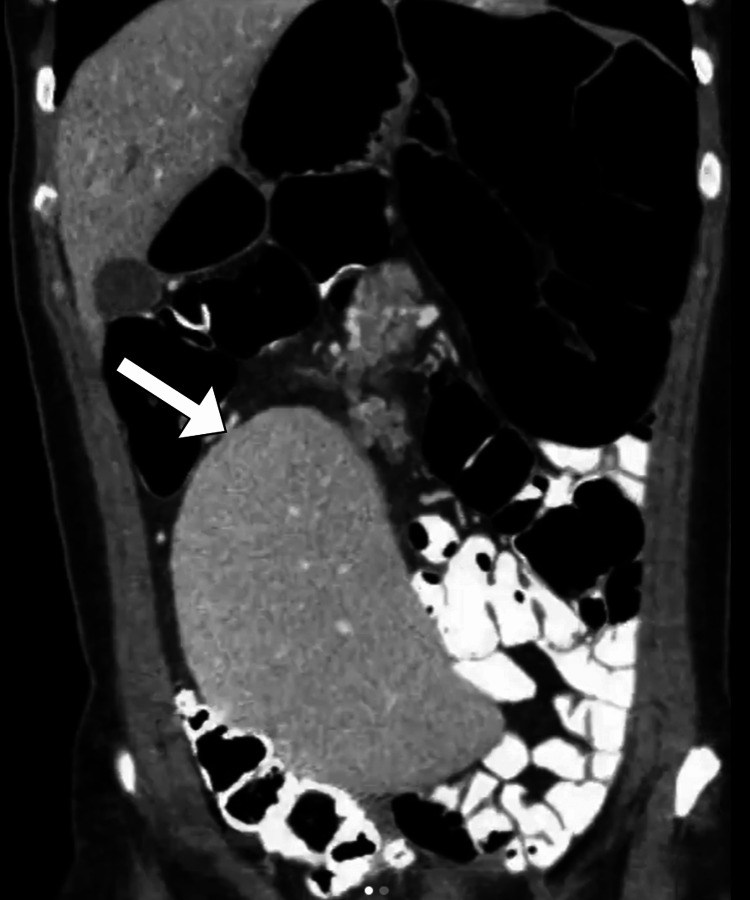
Coronal CT image of the abdomen shows a well-defined mass in the right hemiabdomen and pelvis (arrow) with a similar density of splenic tissue.
